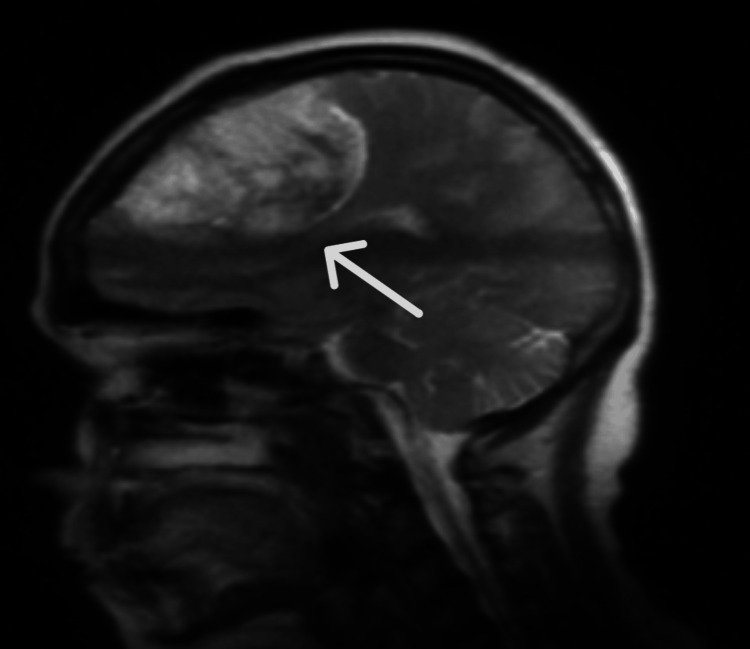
A T2-weighted sagittal MRI image showing a well-defined mass in the frontal region with its base to the periphery of the meninges (arrow). MRI, Magnetic Resonance Imaging
